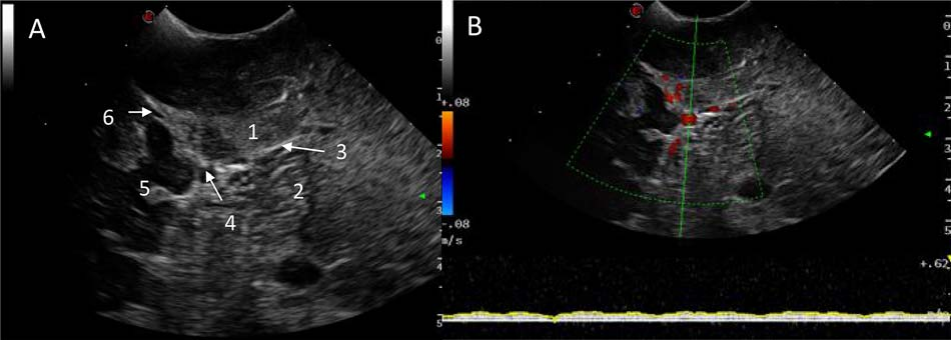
IoUS initial assessment; (A) B-mode image of the surgical field; 1, supratentorial tumour; 2, infratentorial tumour; 3, tentorium; 4, straight sinus; 5, contralateral occipital lobe; 6, falx; (B) triplex Doppler of the straight sinus: combined 2D image with overlay of the colour and pulse wave Doppler demonstrating unidirectional flow from the vein of Galen and the tributary veins of the tentorium.
